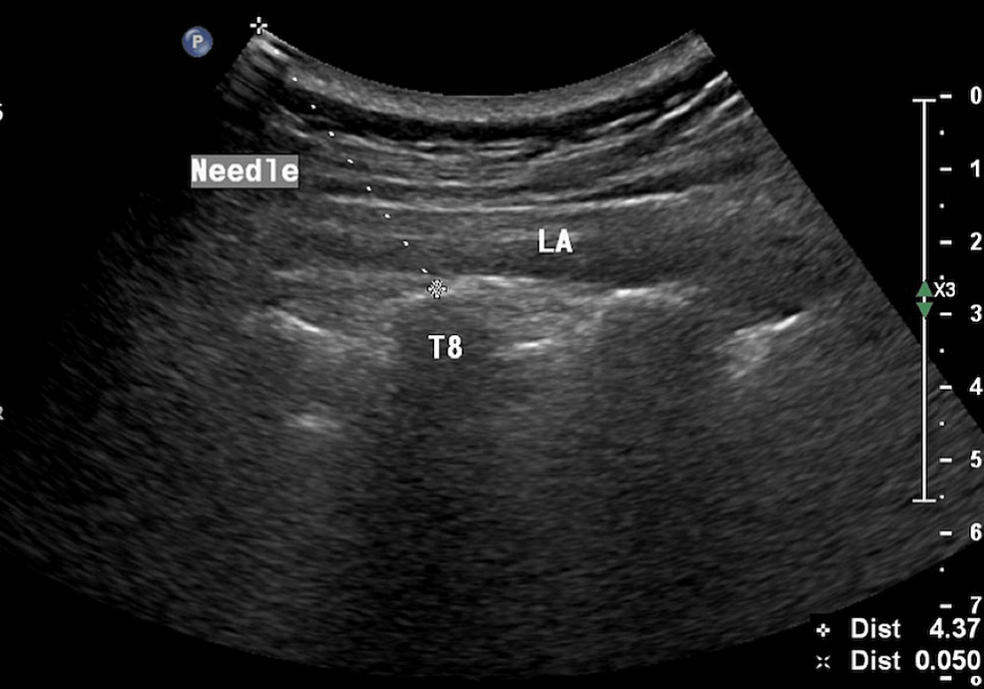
Ultrasound image showing the opening of the plane and spread of local anesthetic LA: local anesthetic.
